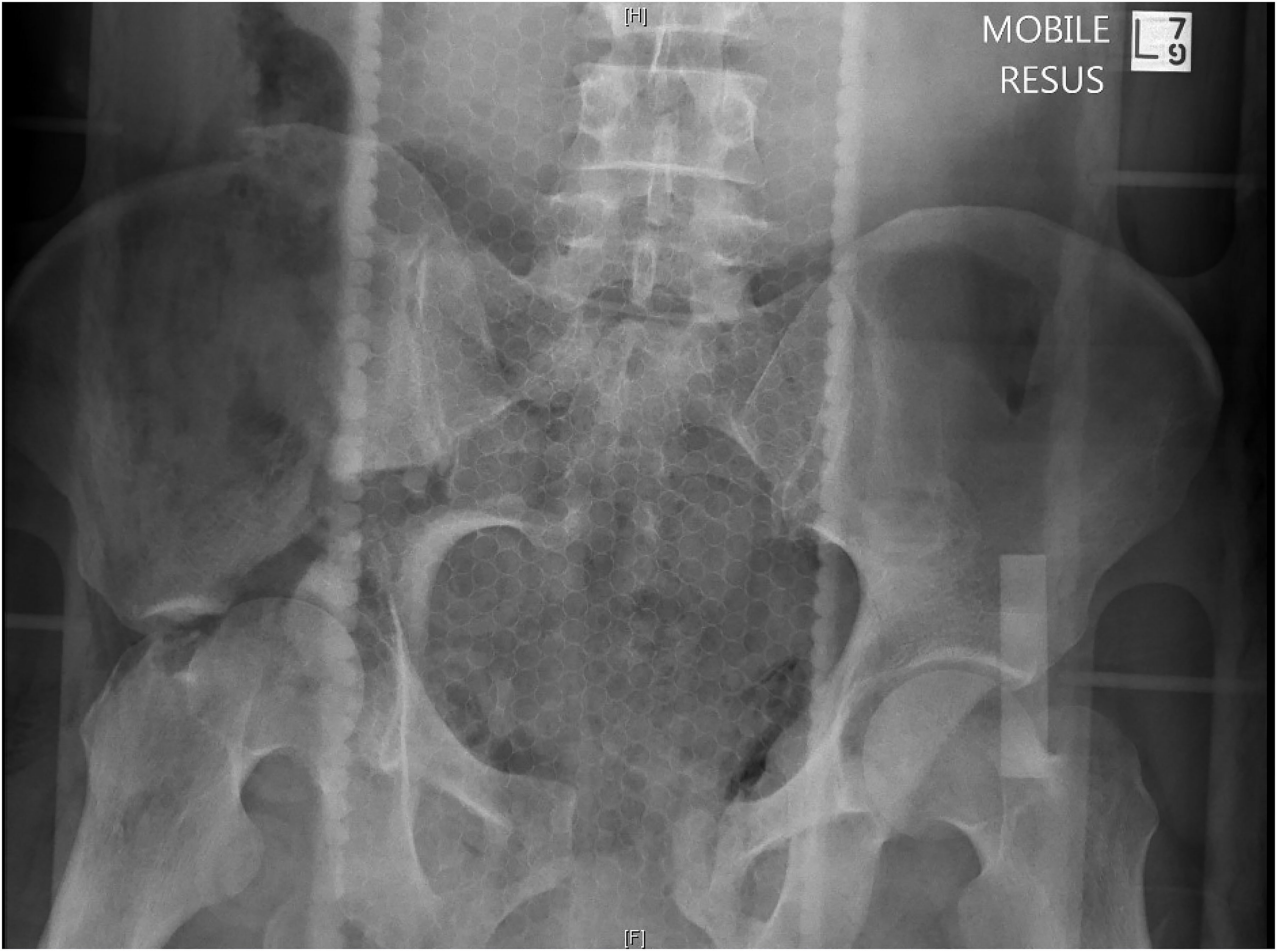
Pelvic x-ray in Emergency Department- 38-year-old male, motor bike vs. car.
